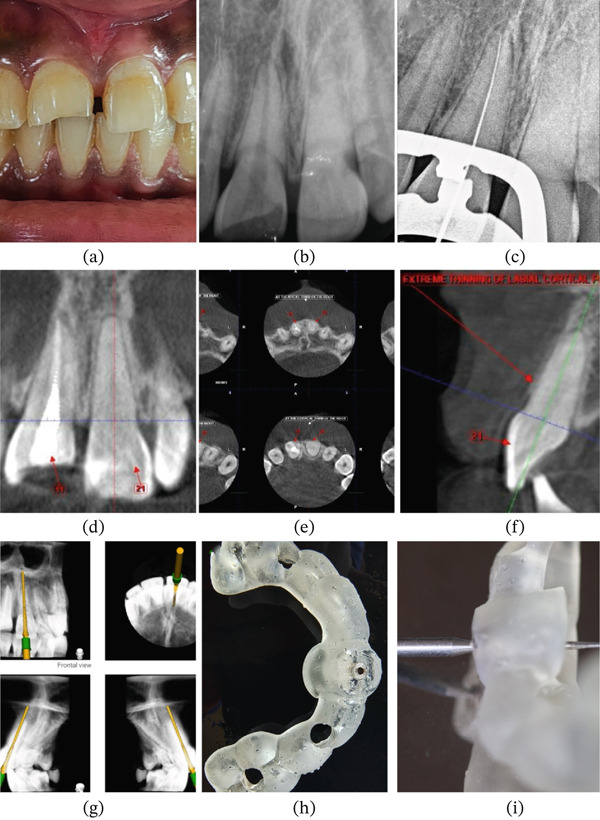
(a) Preoperative clinical image, (b) preoperative radiograph, (c) endodontic treatment of Tooth 11, (d–f) CBCT images, (g) planning of drill path using implant planning software, (h) fabricated endoguide, and (i) drill.
